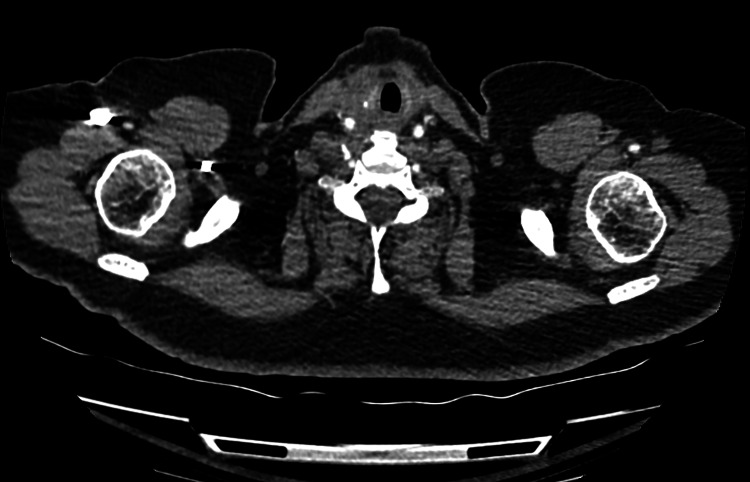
CT neck with contrast scan performed post total thyroidectomy and three cycles of R-CHOP chemotherapy, demonstrating marked regression of the previously visualized thyroid lesion and resolved airway compromise.
